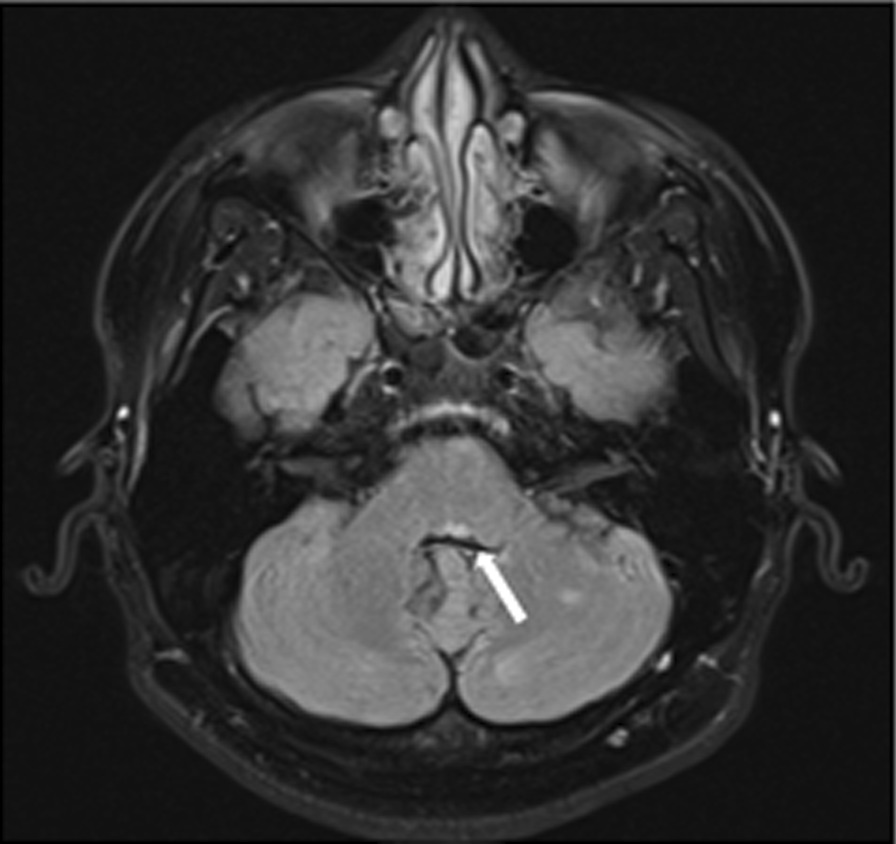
MRI brain T2 FLAIR showing hyperintense lesion (white arrow) over left pontine tegmentum causing left eight-and-a-half syndrome in this patient
